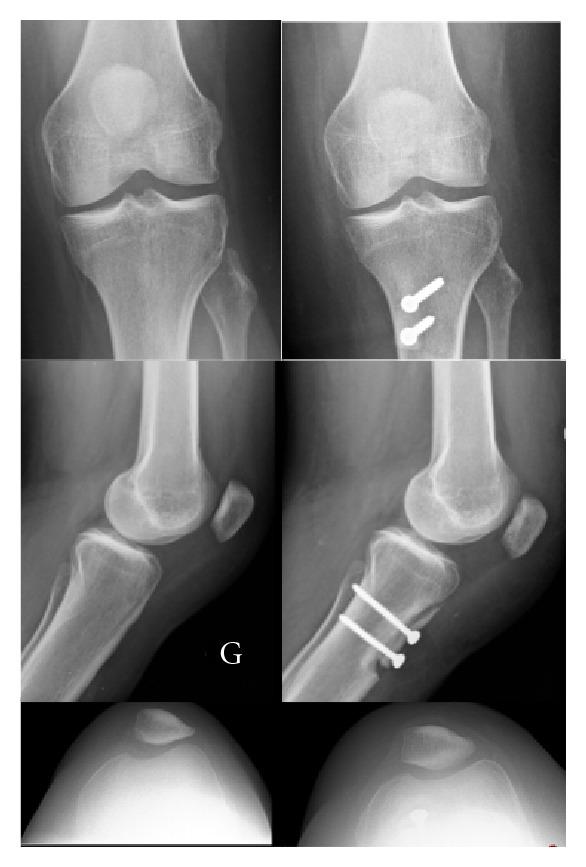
Pre- (left) and postoperative (right) radiograph of the left knee after medial and distal tibial tubercle transfer and vastus medialis plasty. The patella is better centred.
